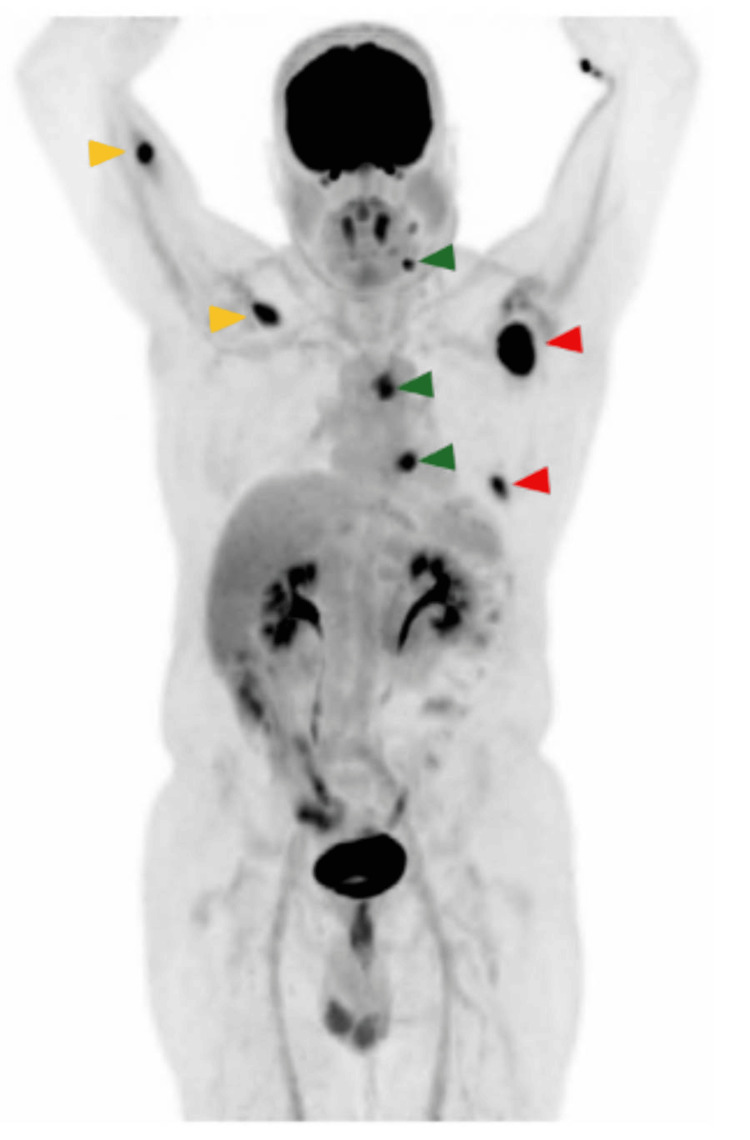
MIP image from 18F-FDG PET/CT The study reveals hypermetabolic cervical and mediastinal lymph nodes (green arrowheads), skeletal muscle metastases involving the right pectoralis major and triceps brachii muscles (yellow arrowheads), and osseous metastatic lesions (red arrowheads), consistent with disseminated metastatic disease. MIP: maximum intensity projection; ^18^F-FDG PET/CT: ^18^F-fluorodeoxyglucose
